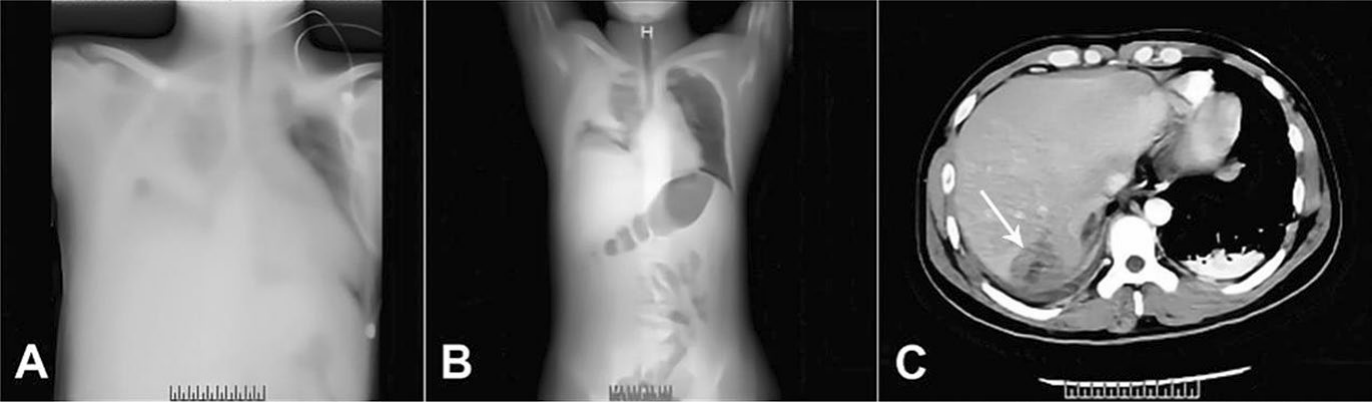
Chest X-ray and computed tomography images of patient #4. a Infected lesions in both lungs with bilateral pleural effusion, partially encapsulated. Inadequate expansion of lung tissue at the fluid surface and solid lung changes near the fluid surface. b Marked dilatation of the small intestine and acute intestinal obstruction due to inflammatory irritation. c Liver abscess (Indicated by a white arrow)
